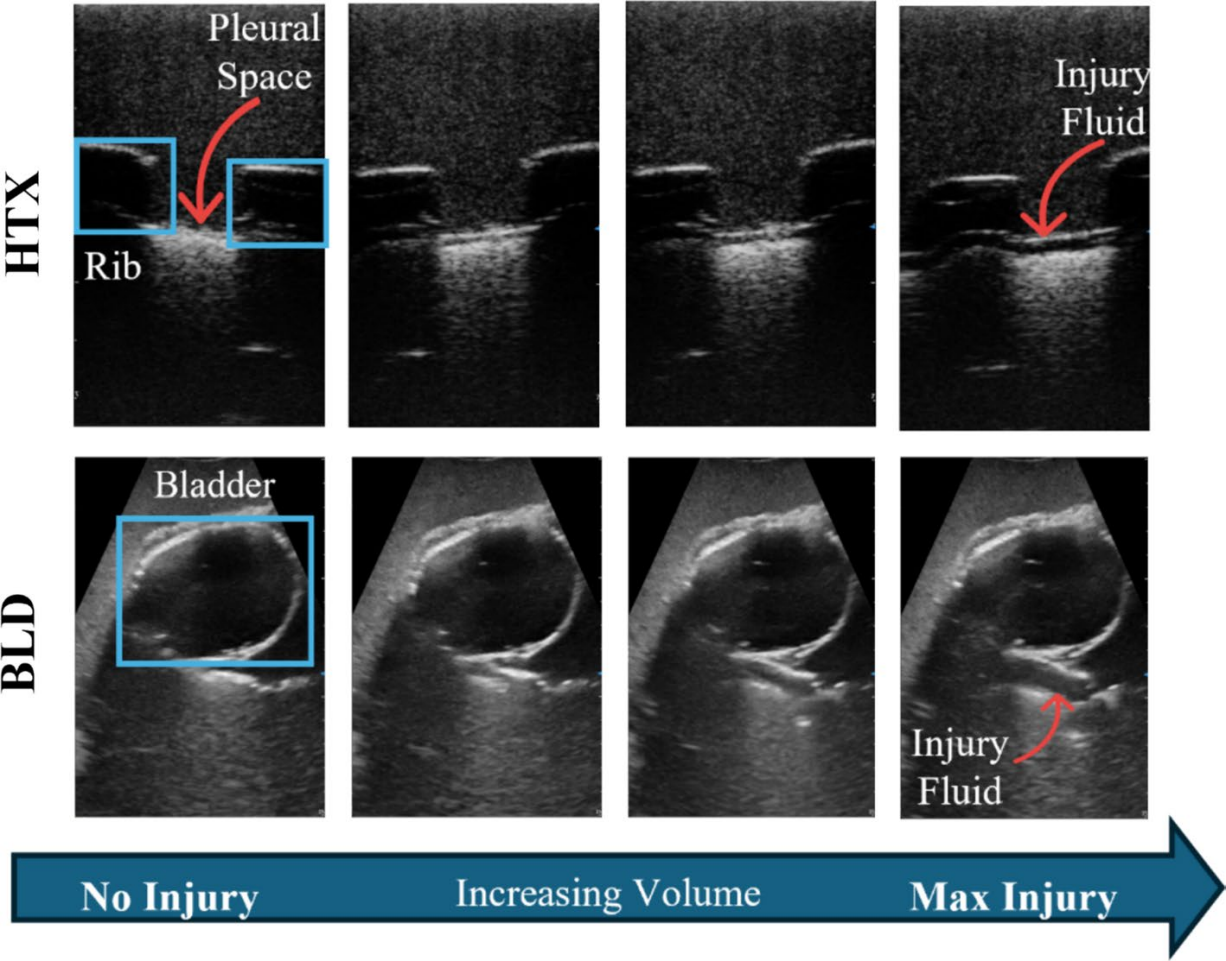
Annotated representative B-mode ultrasound images for the HTX and BLD scan points showing increasing injury volume. Images go from no injury to a full injury from left to right
